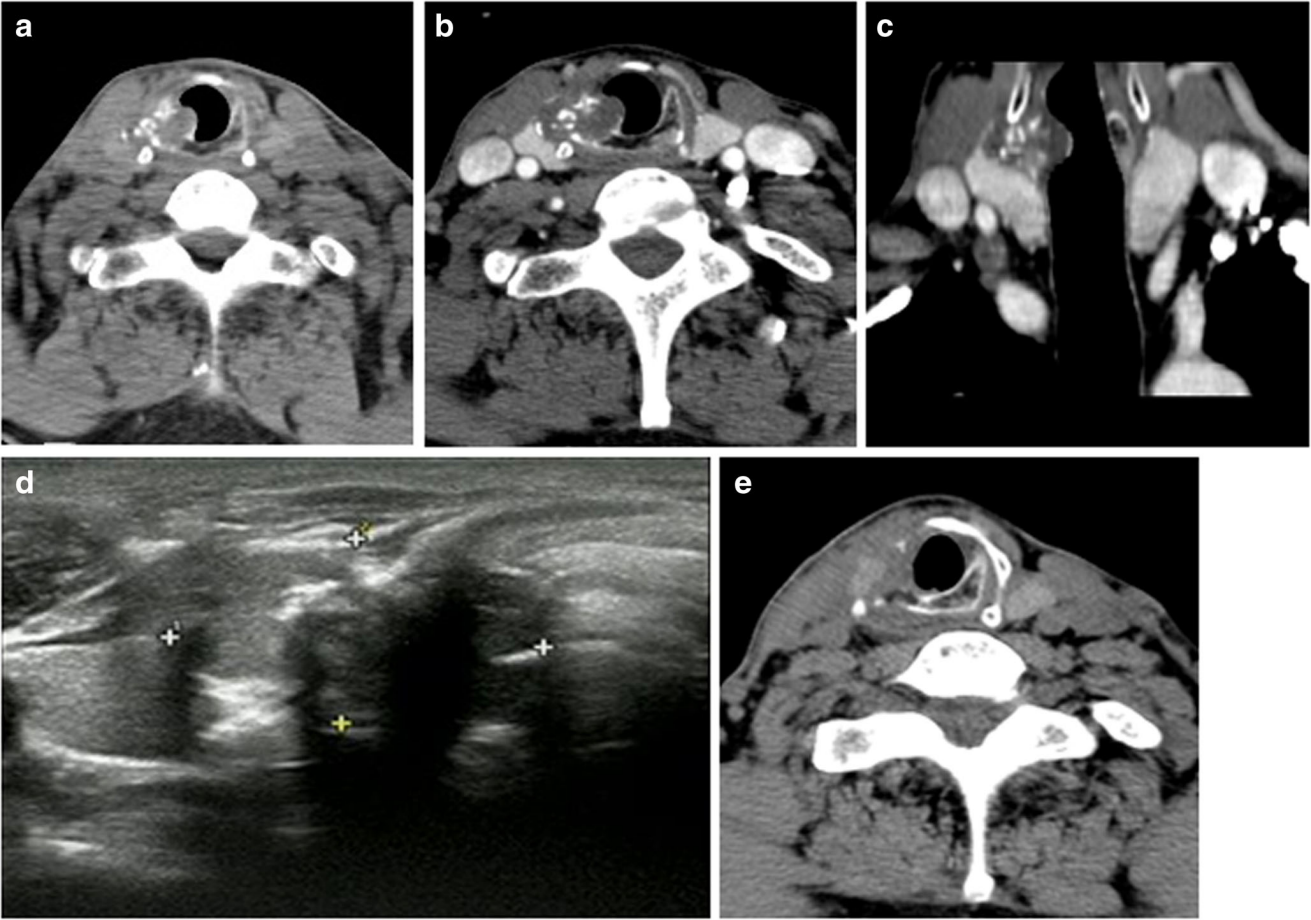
a Axial computed tomography shows a cricoid cartilage tumor at the conjunction of the arch and lamina on the right side. The mass contains calcifications and extends intraluminally and extraluminally. b There was no significant contrast enhancement. The mass compressed the right lobe of the thyroid. c Coronal reformatted image reveals the mass extending intraluminally and extraluminally, causing tracheal narrowing and compression of the thyroid gland. d Ultrasound reveals a hypoechoic mass and multiple calcifications with shadows. e Postoperative computed tomography shows no residual lesion
